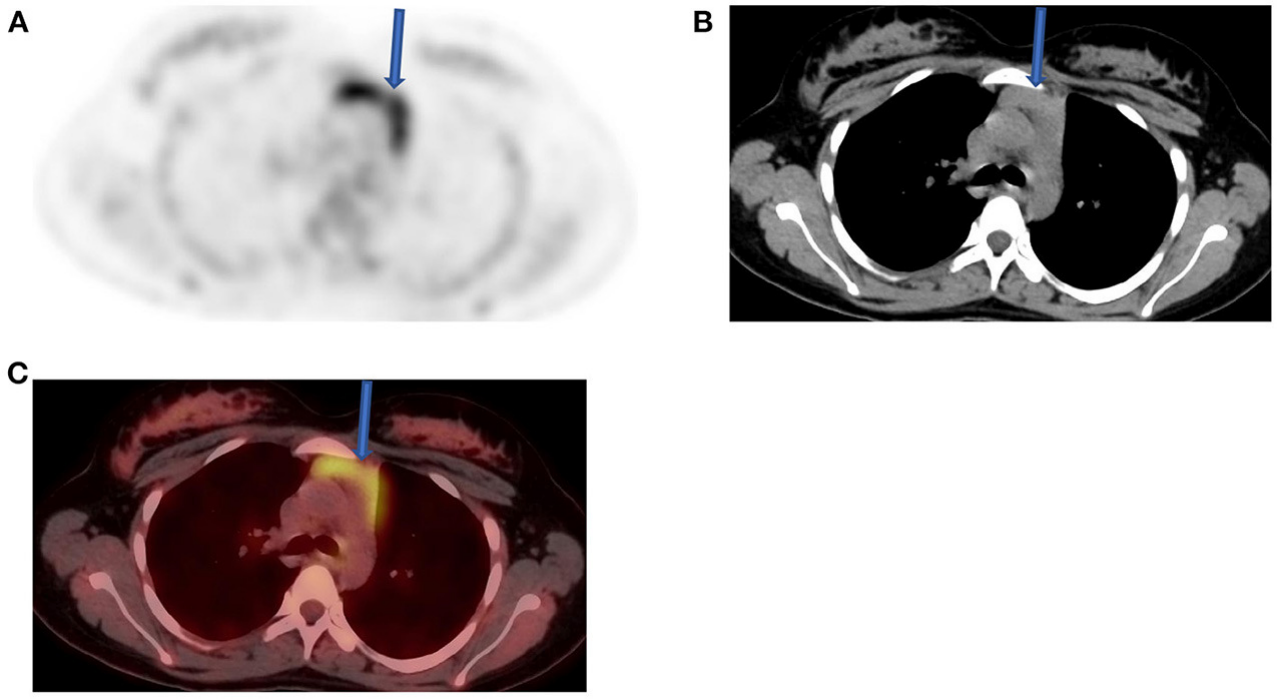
Thymus activity from a 12-year-old female who was diagnosed with Hodgkin's lymphoma (HD) from cervical lymph nodes. She was referred for a staging, and FDG/PET image (A) shows normal uptake in the thymus (solid arrow) with the expected inverted “V” shape that is consistent with a variant of normal. Both CT (B) and fused PET-CT (C) confirmed the nature of visualized change in the anterior mediastinum.
